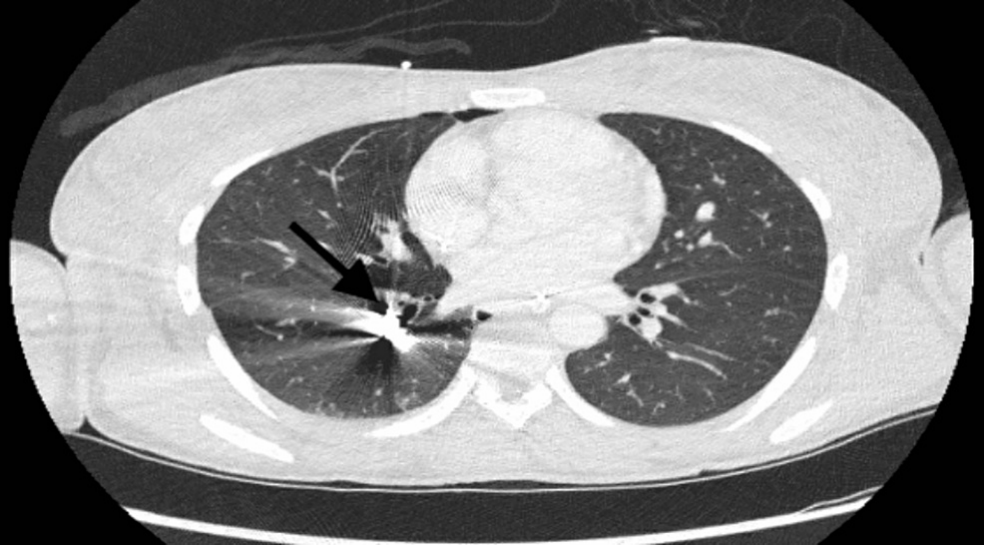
Axial CT chest: streak artifact from bullet within the right lower lobar pulmonary artery. No evidence of pulmonary laceration, contusion, soft tissue injury, or subcutaneous emphysema to suggest direct thoracic injury.
